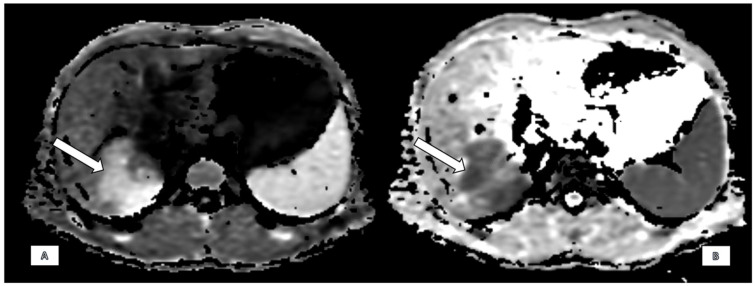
Diffusion-Weighted Imaging (DWI): There is marked diffusion restriction with low apparent diffusion coefficient (ADC) values (A,B).
